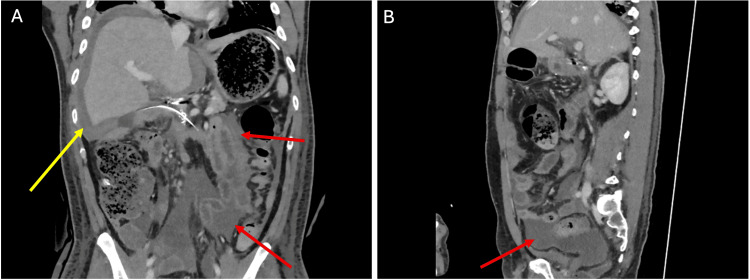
CT images day 18 postoperatively to investigate recurrent fevers and rising inflammatory markers (June 2020) CT imaging in (A) coronal and (B) sagittal views with portal venous contrast enhancement demonstrating intra-abdominal fluid (red arrows), predominantly surrounding the liver (yellow arrow). CT, computed tomography.
